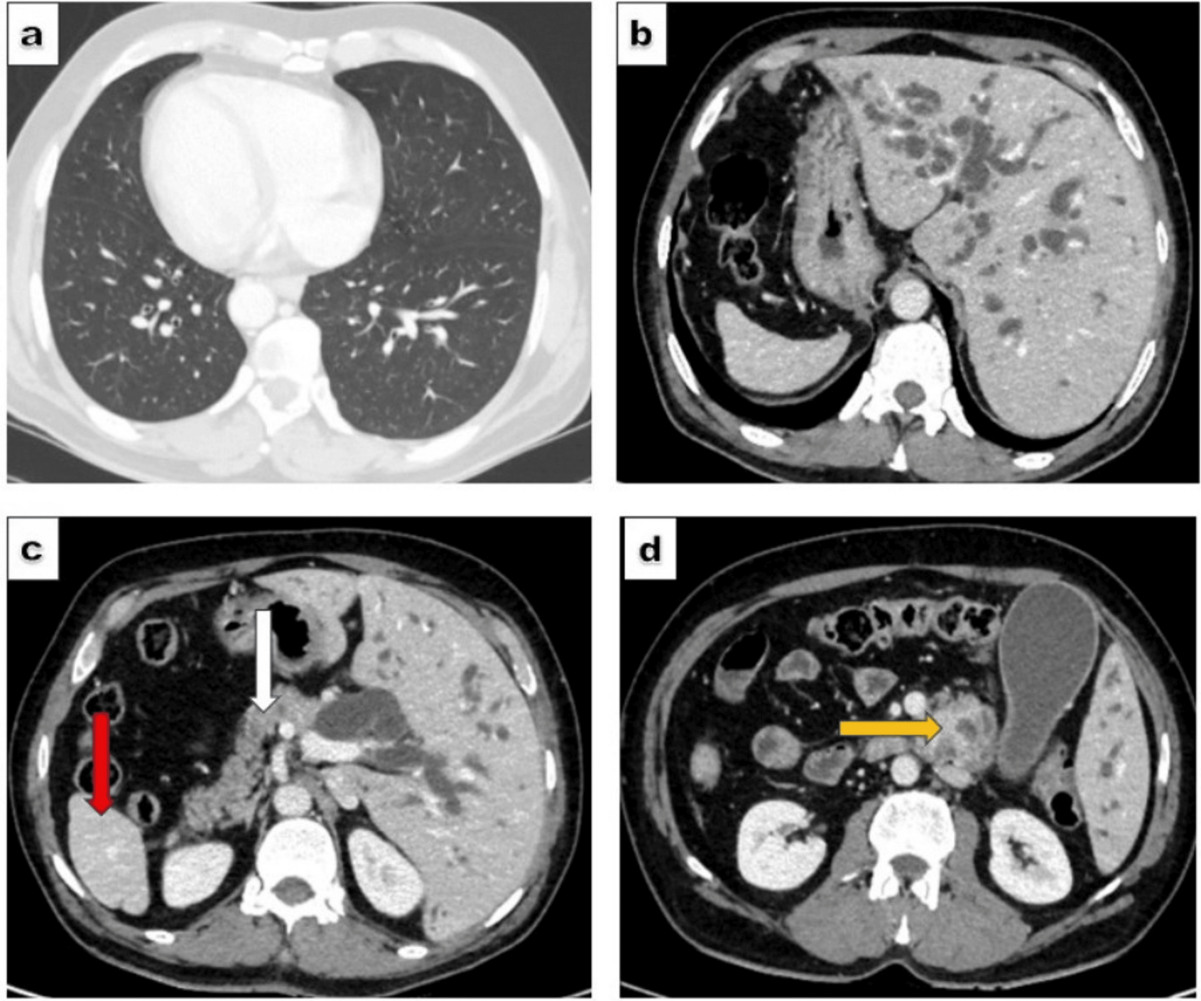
Computed tomography scan images of reversed anatomy. (a) Dextrocardia, (b) Liver on the left side, whereas the stomach lies on the right side, (c) Pancreas (white arrow) and spleen (red arrow) rotated on the right side, (d) Periampullary mass (yellow arrow).
